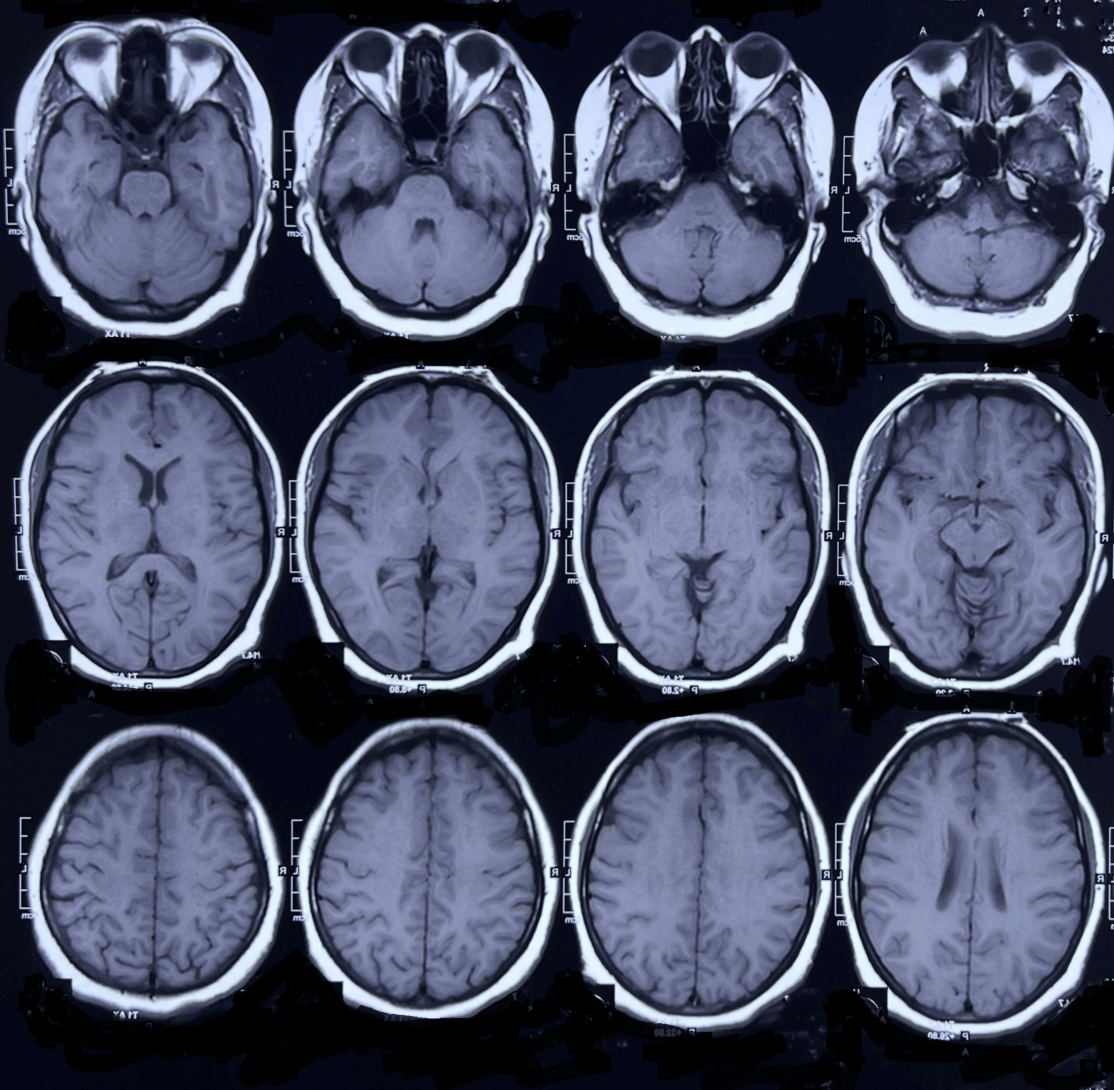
Normal MRI of of the brain (axial view).
